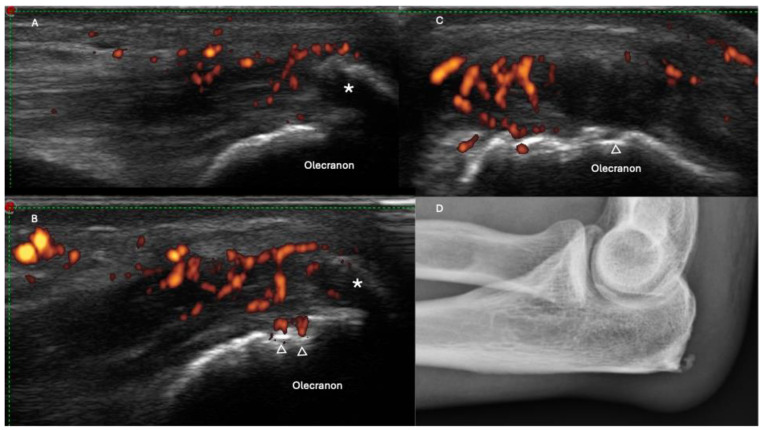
Triceps tendon longitudinal aspect (A–C) showing inflammatory changes, such as thickening, hypoechoic appearance, and the presence of power Doppler within 2 mm of the cortical bone. Damage findings such as enthesophytes (marked with *) and bone erosion (marked with Δ) can also be seen, in correlation with enthesophyte findings in an elbow X-ray (D).
